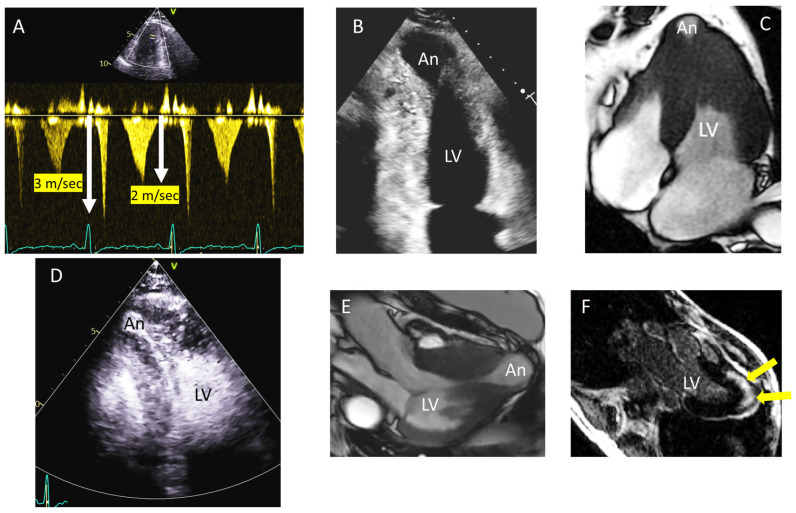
Apical HCM with aneurysm. Typical Doppler patterns, contrast echocardiography and cardiac magnetic resonance (CMR) aid in the detection of apical aneurysms. (A) A typical continuous Doppler void pattern (early systolic high velocity towards the LV cavity—3.3 m/s, and early diastolic paradoxical flow—2.2 m/s, known as the ‘lobster claw’ abnormality). (B) Two chamber view with a clear detection of the aneurysm (An). (C) CMR (cine four-chamber view) showing a small aneurysm and (D) same case as demonstrated by contrast echocardiography. (E) CMR (cine three-chamber view) of a larger aneurysm. (F) CMR (late gadolinium enhancement three-chamber view sequence) showing transmural apical scarring (arrows). An: aneurysm.
